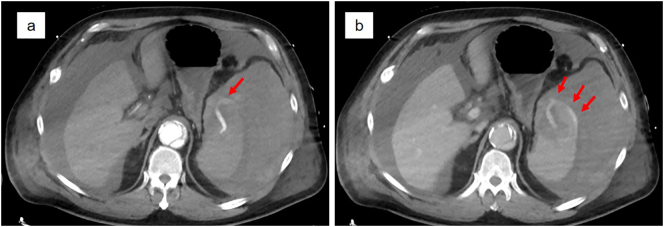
Contrast-enhanced computed tomography demonstrates intraabdominal hemorrhaging, hematomas around the spleen, and extravasation of contrast medium within the spleen (arrows) (a: arterial phase, b: equilibrium phase).
